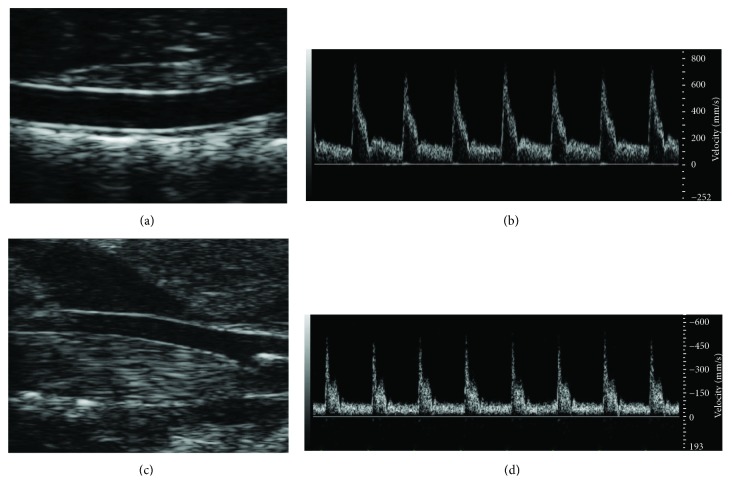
Abdominal aorta ultrasound scan. (a) B-mode image sequence was used to derived diameter and distension. (b) PW-Doppler signal was used to plot diameter-flow velocity loop thus evaluating PWV_abd_. Aortic pulse pressure was derived from PWV_abd_ and distension measurements. Furthermore, blood flow velocity signal was used to derive wall shear rate measurements. Carotid artery ultrasound scan. (c) B-mode image sequence was used to derived diameter and distension. (d) PW-Doppler signal was used to plot diameter-flow velocity loop thus evaluating PWV_car_. Furthermore, blood flow velocity signal was used to derive wall shear rate measurements.
